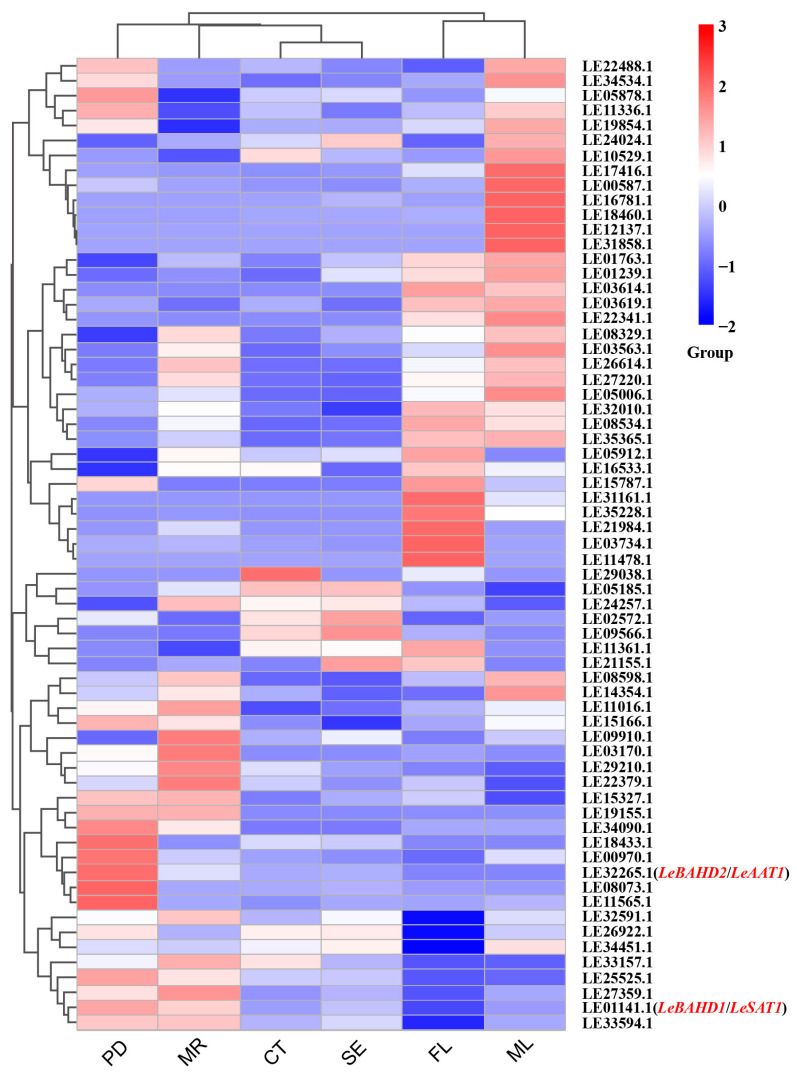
Expression patterns of *LeBAHDs* in different tissues of *L. erythrorhizon* seedlings based on transcriptome data. MR: mature roots; PD: root periderm; FL: flowers; ML: mature leaves + stems; CT: root cortex; SE: root stele.
